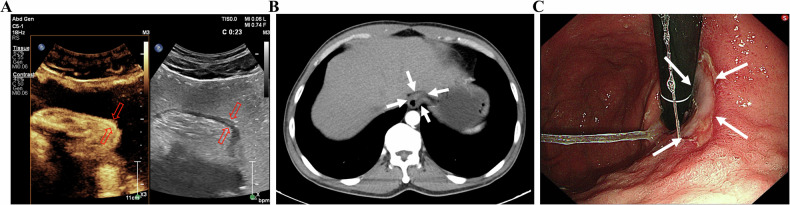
A An approx 55-year-old man underwent gastric double contrast-enhanced ultrasonography and oral contrast ultrasonography, revealing a subserous layer significantly enhanced in the arterial phase (left) and a low echo invaded the subserosal layer (cT3) at the cardia (right), respectively. The hierarchical structure of the gastric wall was unclear. The mucosa presented as rough, and the strong echogenic zone of the serosa was not smooth. B The tumor showed local thickening and enhancement, indicating invasion to the subserosal layer (cT3) of the cardia and the mall curvature side of the gastric body on enhanced computed tomography. C Electronic gastroscopy found a large and deep ulcer on the lesser curvature of the cardia
